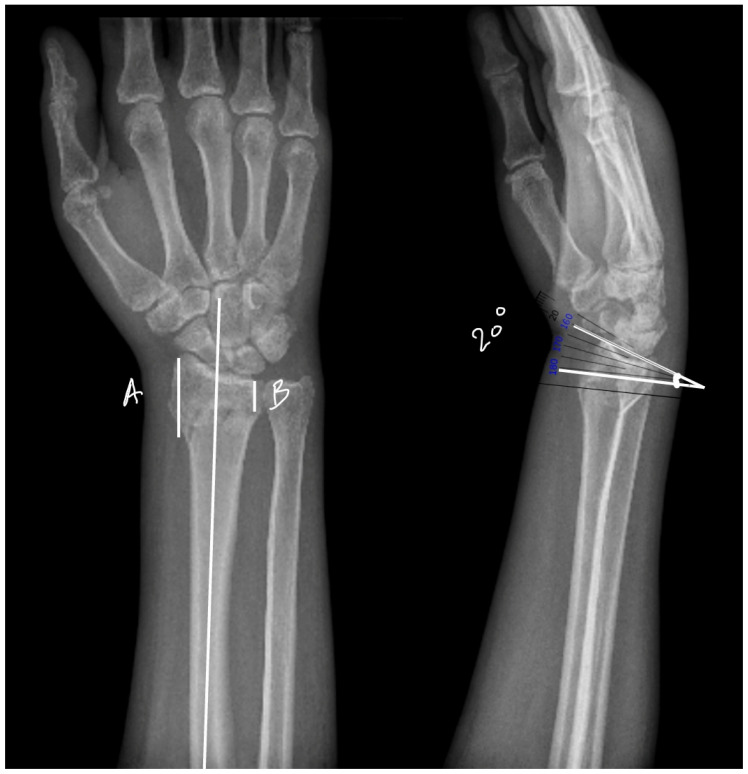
Dorsally displaced distal radius fracture measurements on anteroposterior (AP) and lateral radiographs. On AP views, we can calculate (A) lateral side measurement of the fracture line distance from the articular surface, and (B) medial side measurement of the fracture line distance from the articular surface. And on lateral views, we can calculate the mean angle between the radiocarpal joint line and fracture line.
